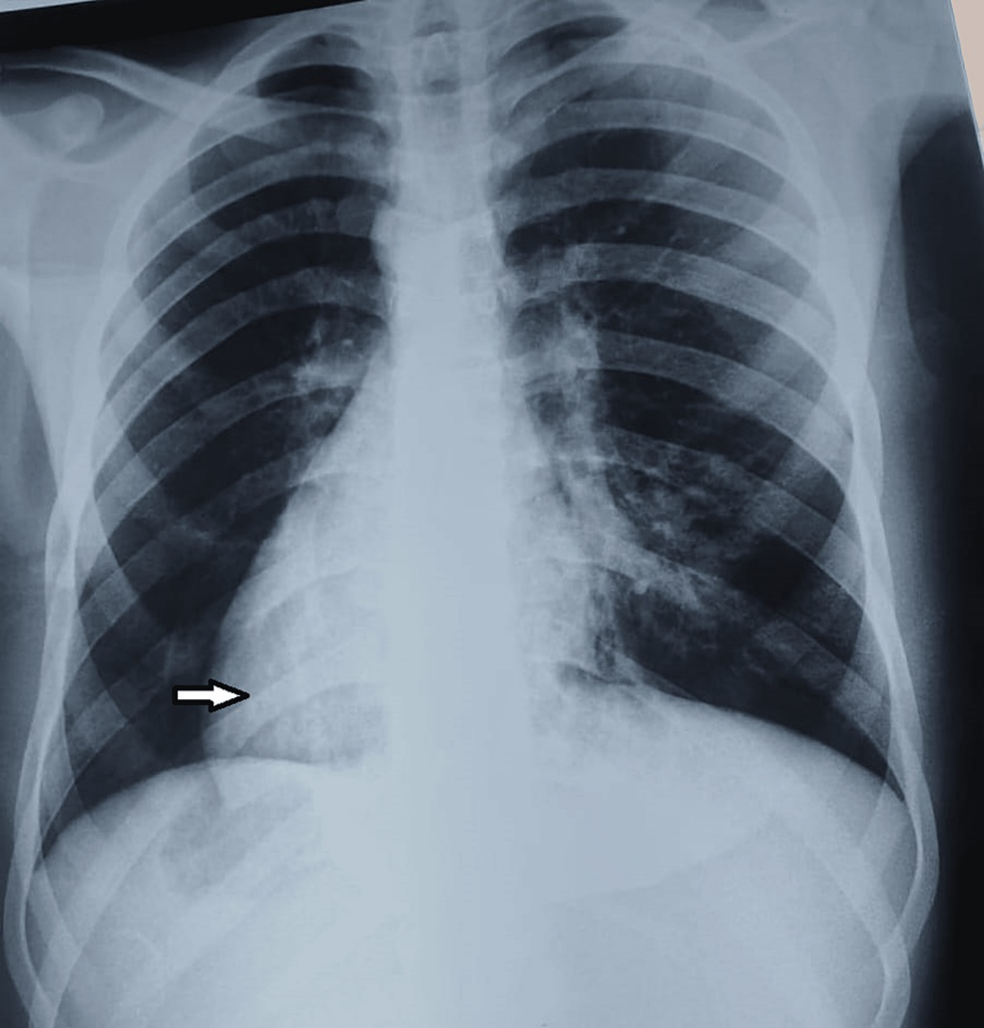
Chest X-ray showing the situs inversus (white arrow), bronchial syndrome, and alveolar focus of the lingula
